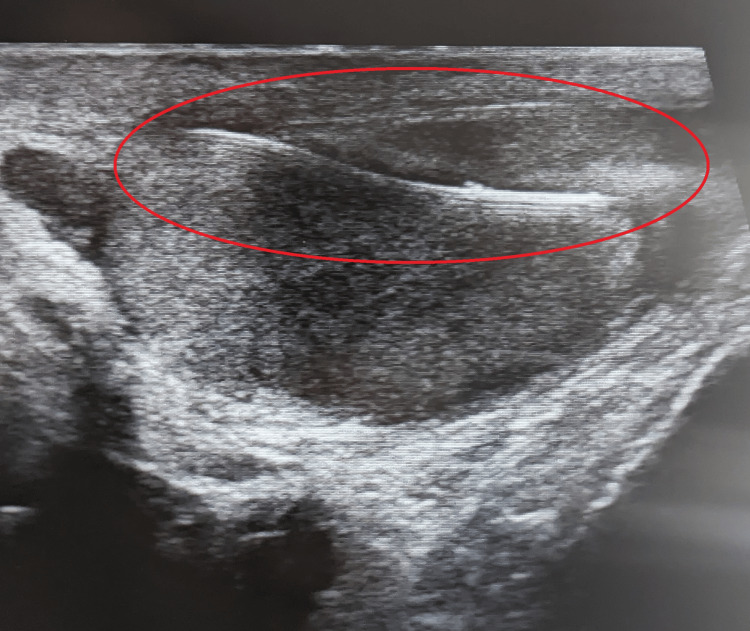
Ultrasonographic visualization of a retained metallic foreign body in the left testis. High-resolution ultrasound demonstrates a linear, hyperechoic structure traversing the testicular parenchyma (red circle). These sonographic features are characteristic of metallic objects and confirm the intratesticular location of the foreign body. Probe alignment and angle were adjusted to optimize visibility.
